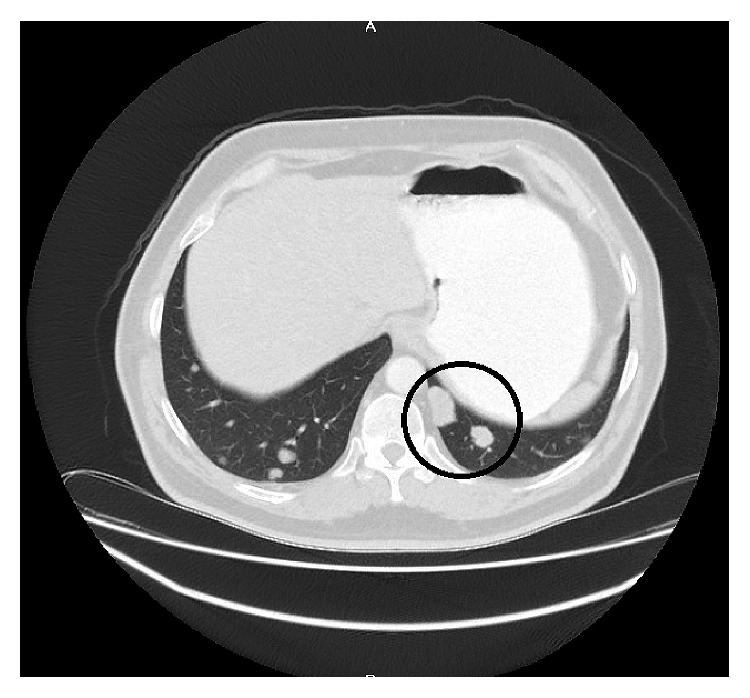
PET scan-correlated CT section of lung nodule at the diagnosis of BRAF-mutant mCRC.
